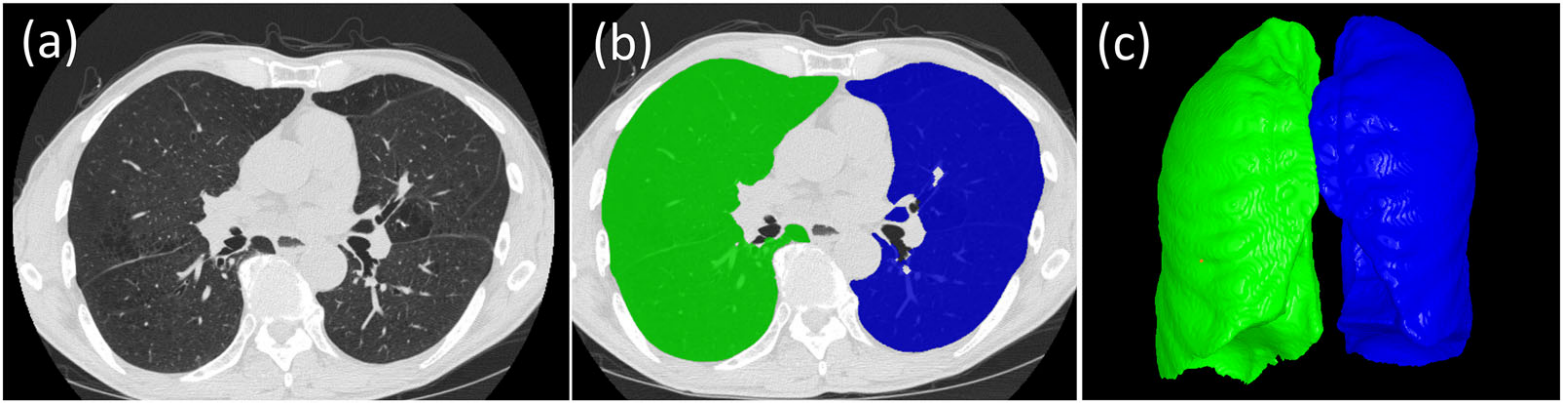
The lung volume segmentation: (a) original computed tomography (CT) images, (b) the segmented right and left lung regions in overlay, and (c) the three‐dimensional (3‐D) visualization of the segmented lungs
